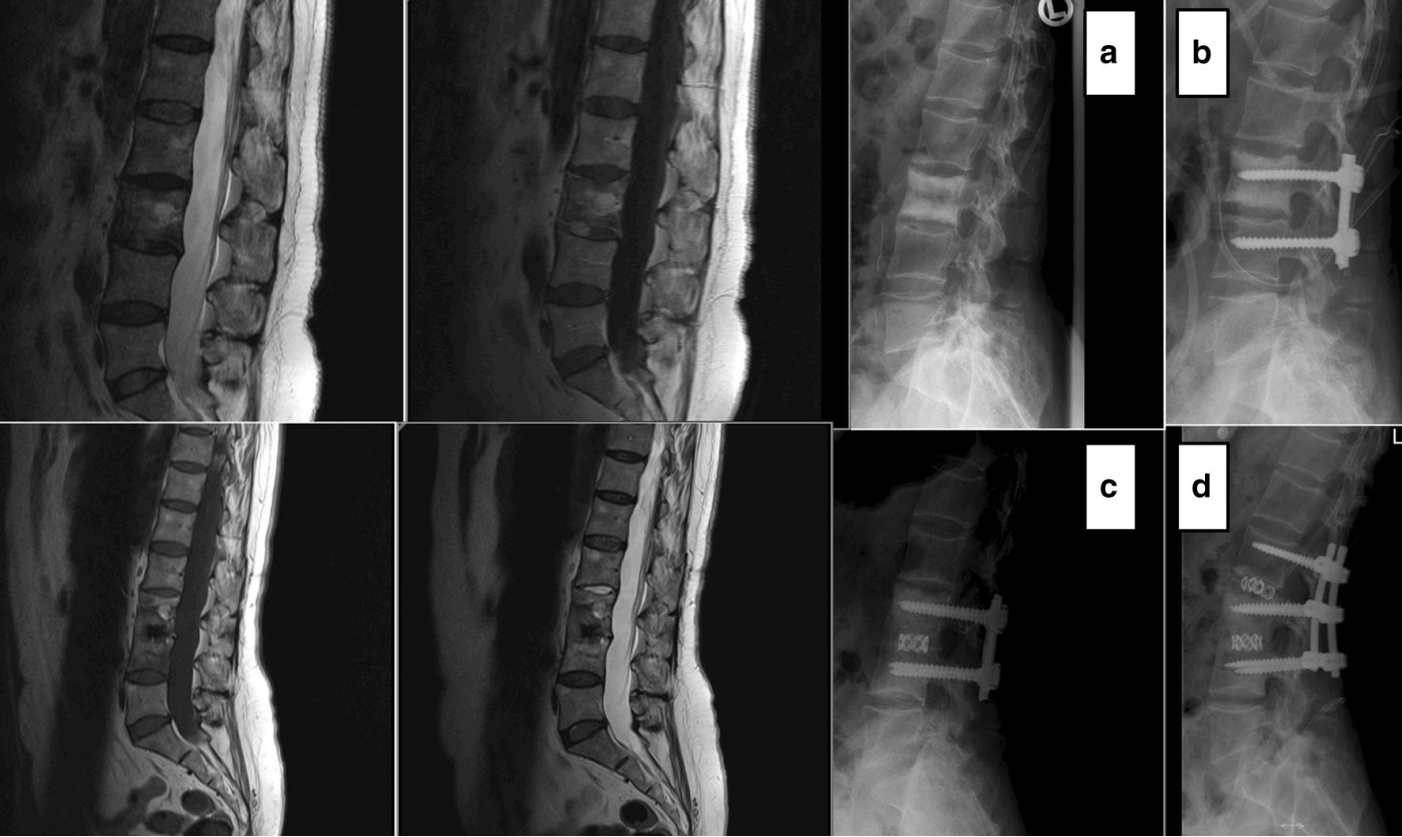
Patient 18: a preoperative MRI and radiographs, b postoperative, c ASI in MRI and radiographs and d after reoperation
